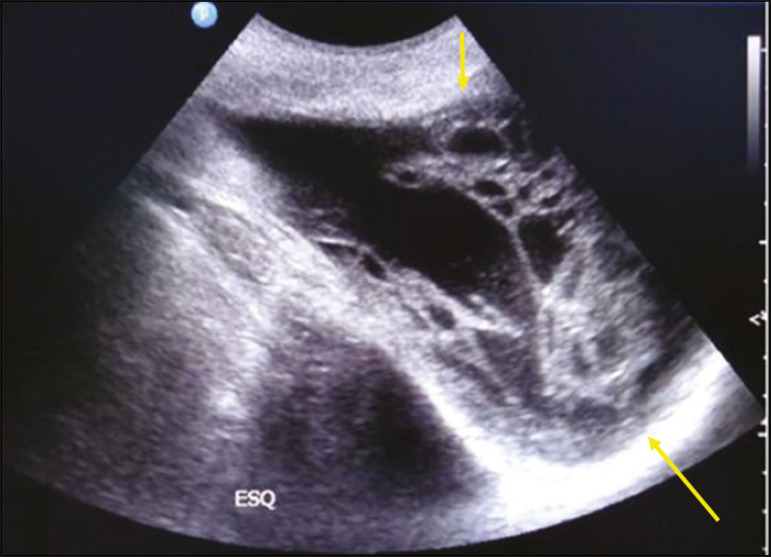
Inguinoscrotal hematoma as a complication of inguinal herniorrhaphy. Ultrasound showing a multilocular formation with heterogeneous, hyperechoic components (arrows).
